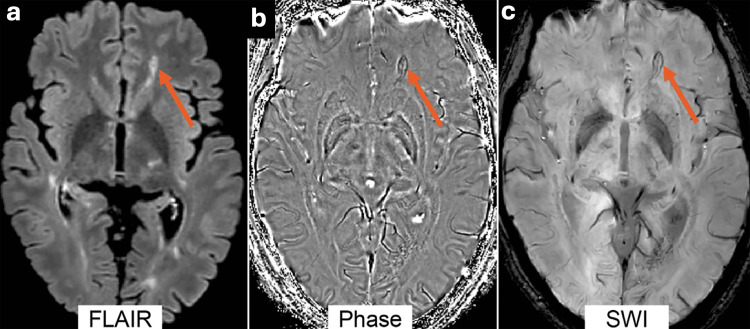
An example of hyperintense lesions shown on (a) FLAIR at 3T. The red arrow indicates one of the hyperintense lesions, which has a hypointense rim on the (b) phase image and (c) SWI. Reproduced from Rocca MA et al [3] under the terms of the Creative Commons Attribution License (CC BY 4.0).
